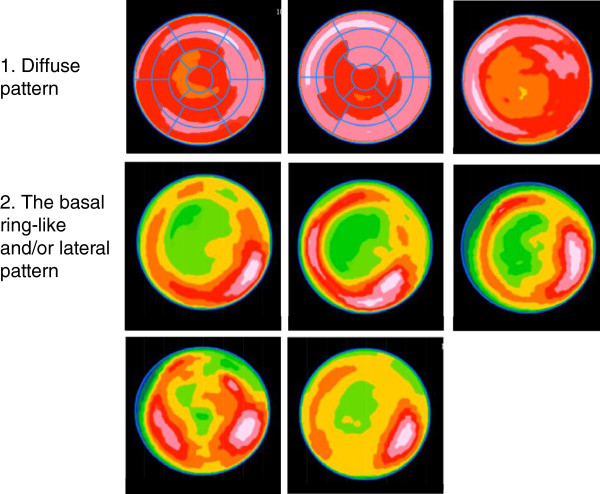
Polar maps of healthy volunteers with myocardial FDG uptake. There were two patterns of myocardial FDG uptake (grade 2, 3, or 4) in healthy volunteers when they had preparation with 12-h fast plus heparin injection (HEP) or long fast (16 h or more, LF). The polar map patterns include (1) a diffuse uptake pattern and (2) a basal ring-like and/or lateral uptake pattern.
